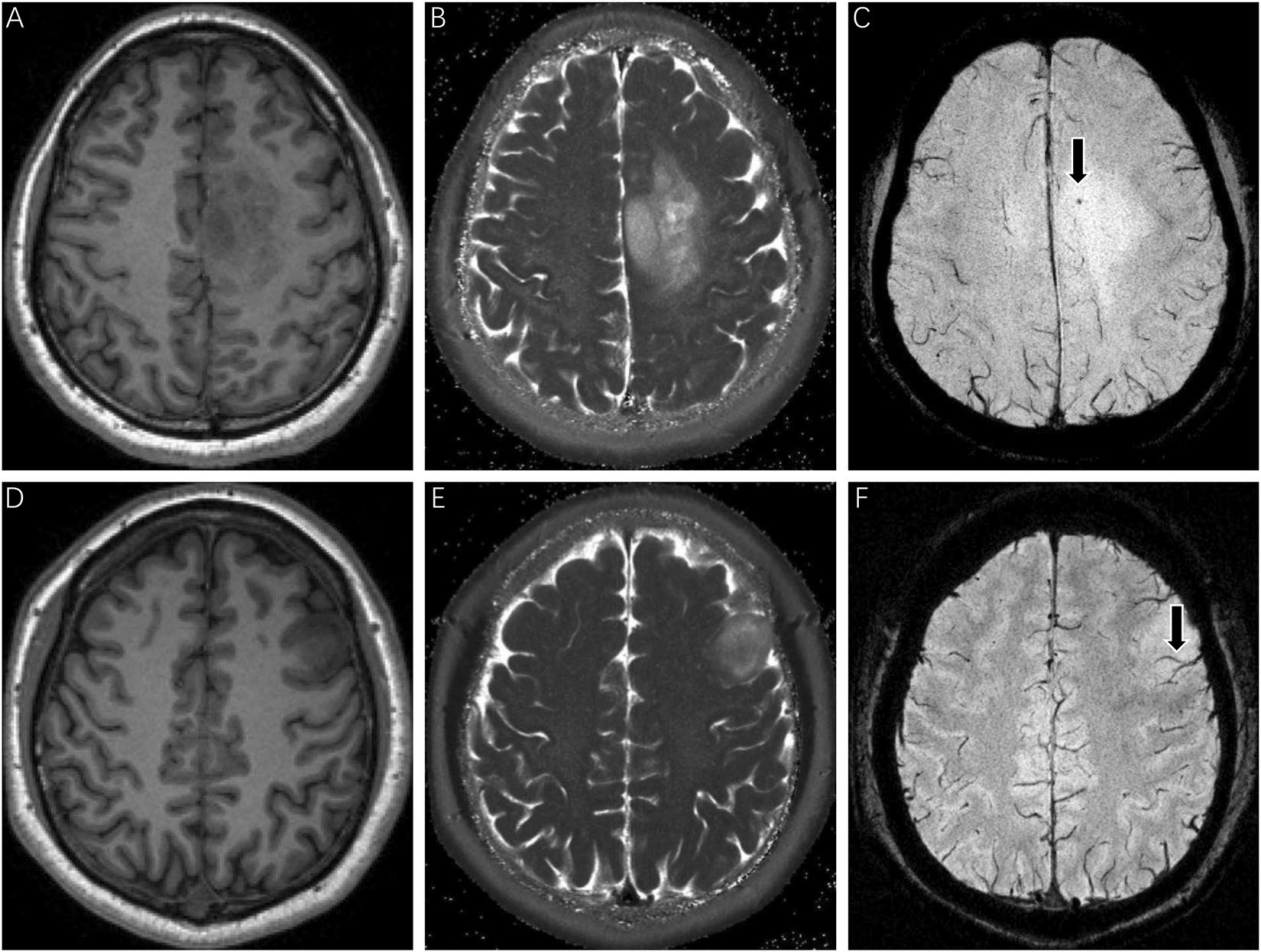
SWI imaging features of gliomas. SWI imaging (C,F) shows significant advantages in identifying microbleeds and microvessels in comparison with T1-weighted (A,D) and T2-weighted (B,E) imaging (as indicated by the arrows).
